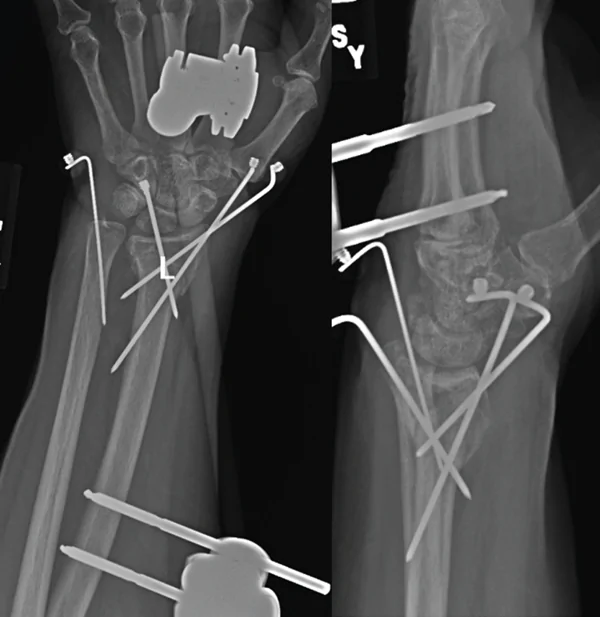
Radiographs at 8 weeks postoperatively demonstrating satisfactory alignment and healing with external fixator and Kirschner wires in place.
